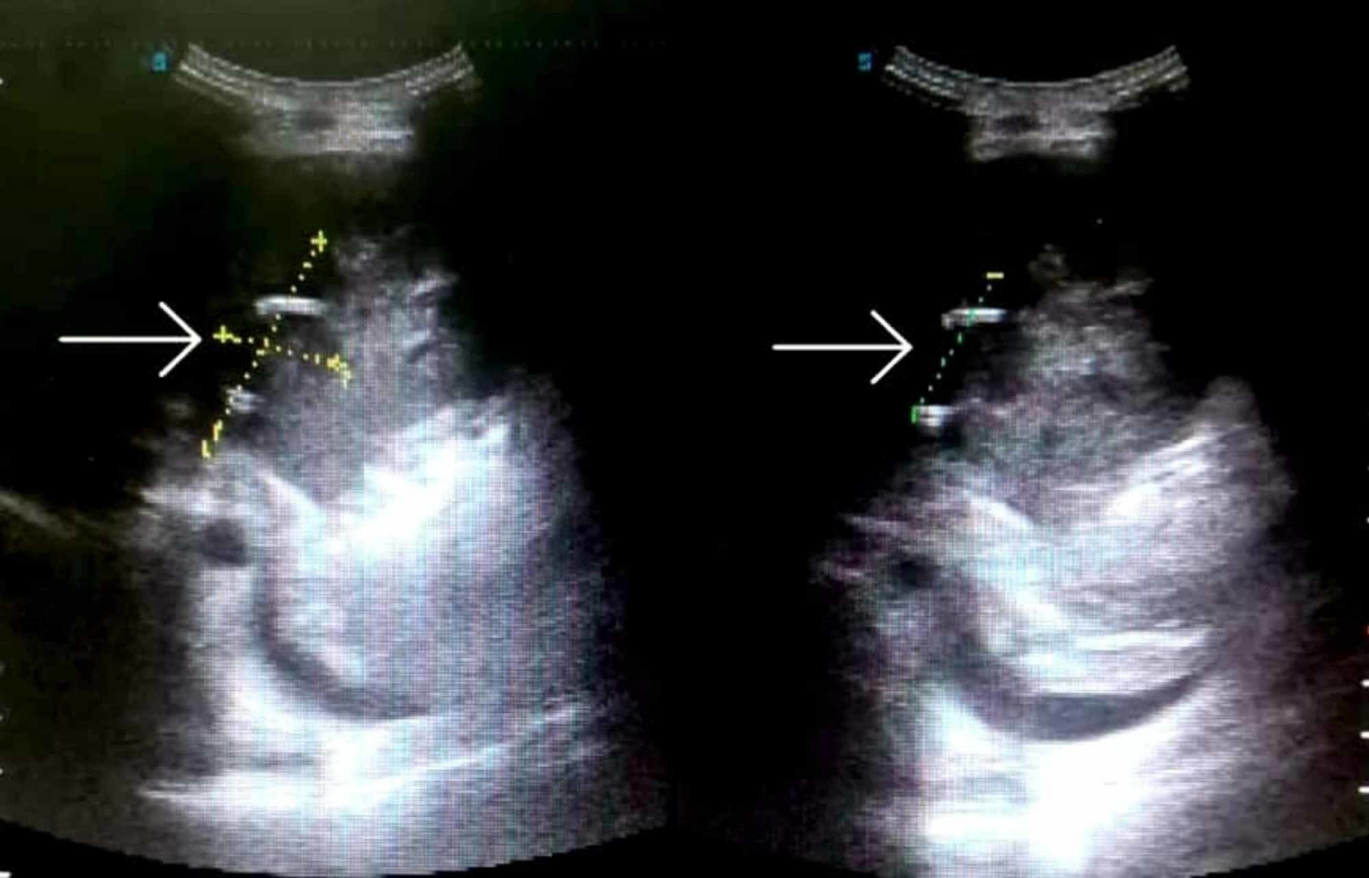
Abdominal ultrasound showing a residual abscess in the right lobe of the liver
